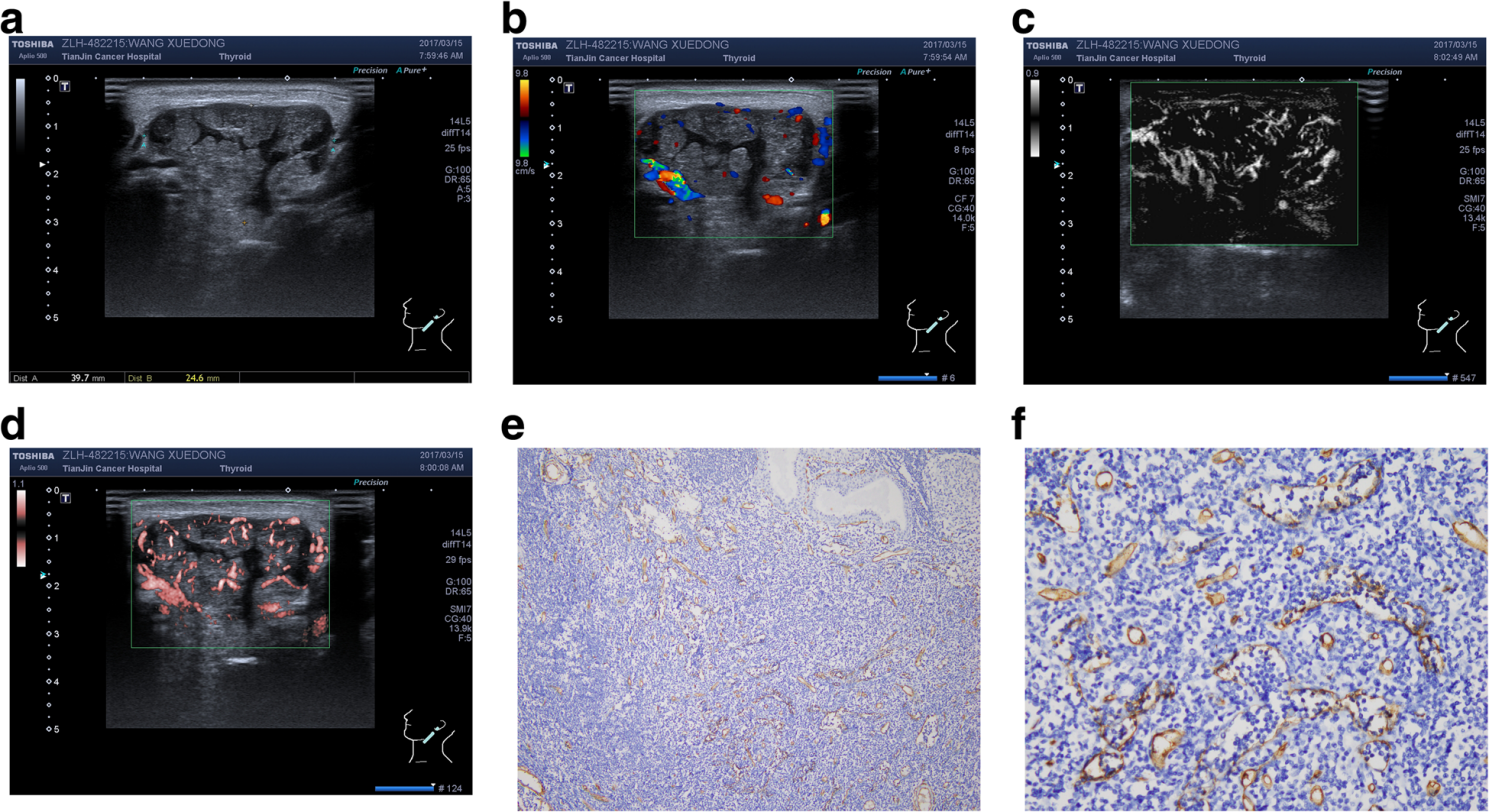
Represent WT case from right parotid gland. a. Conventional ultrasound image; b. CDFI: 3–4 punctate blood flow, Peripheral distribution, Alder 2; c. mSMI and d. cSMI: Mixed distribution, Alder 3. VIby cSMI:7.4; e. MVD by IHC (× 100); f. MVD by IHC: 38 (× 400)
